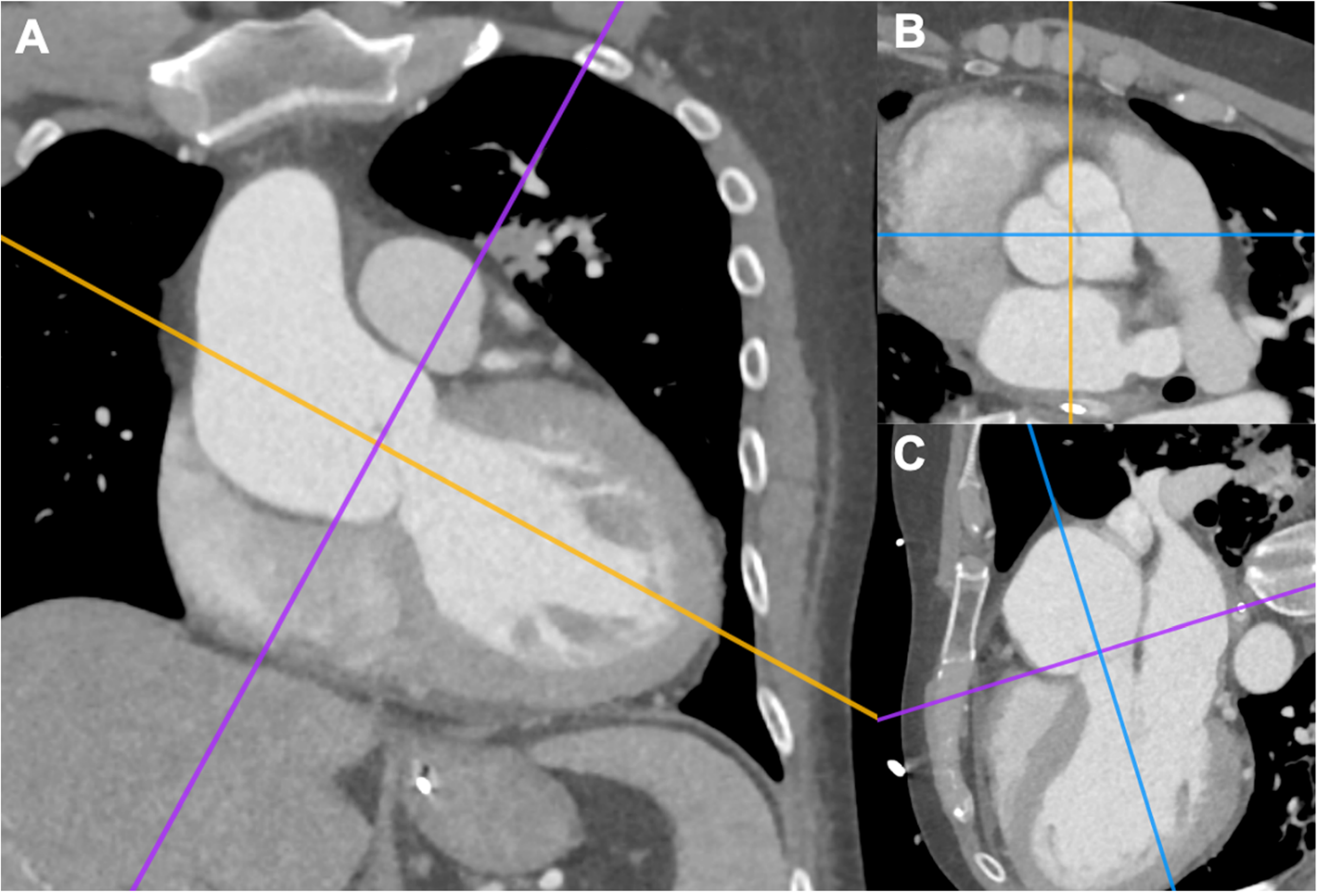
3-dimensional computed tomography reconstruction, with the visualization of the aortic root on a plane perpendicular to the long axis of the aorta (A-C)
